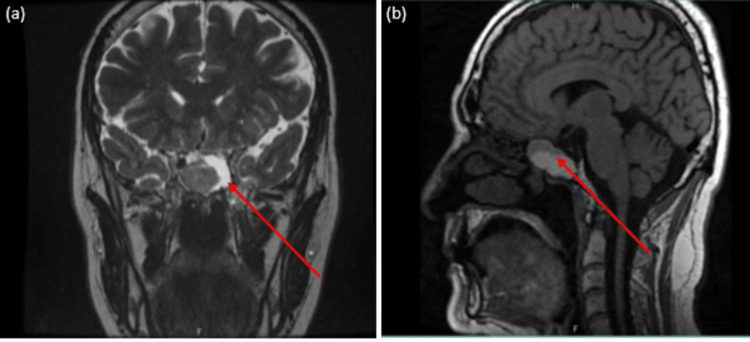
MRI sella with coronal (a) and (b) sagittal cuts showing the large sellar mass (red arrows)
